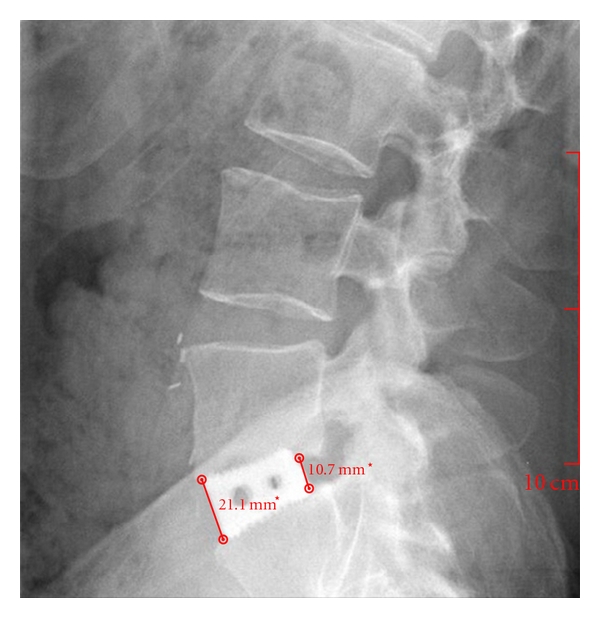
Measurement of digital X-ray.
